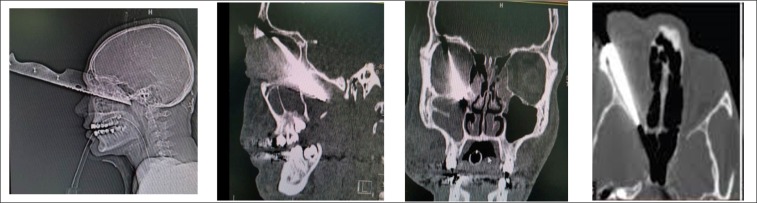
CT scan view shows orbital and skull base involvement
